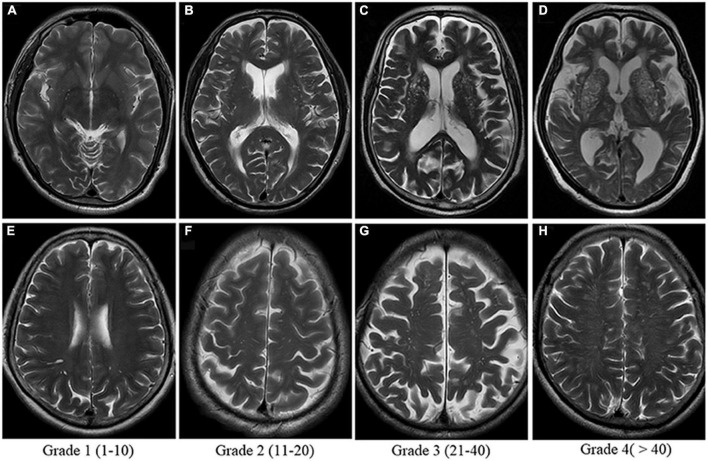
Enlarged perivascular space (PVS) visual score rating as shown on T2-weighted MRI. Grades 2, 3, and 4 are considered abnormally enlarged. Panels (A–D) show severity of enlarged PVS in the Basal Ganglia. Panels (E–H) show severity of enlarged PVS in the centrum semi-ovale. The Basal Ganglia and Centrum Semi-Ovale are currently implicated as the most accurate measures of PVS burden. Image from Yu et al. (2022).
